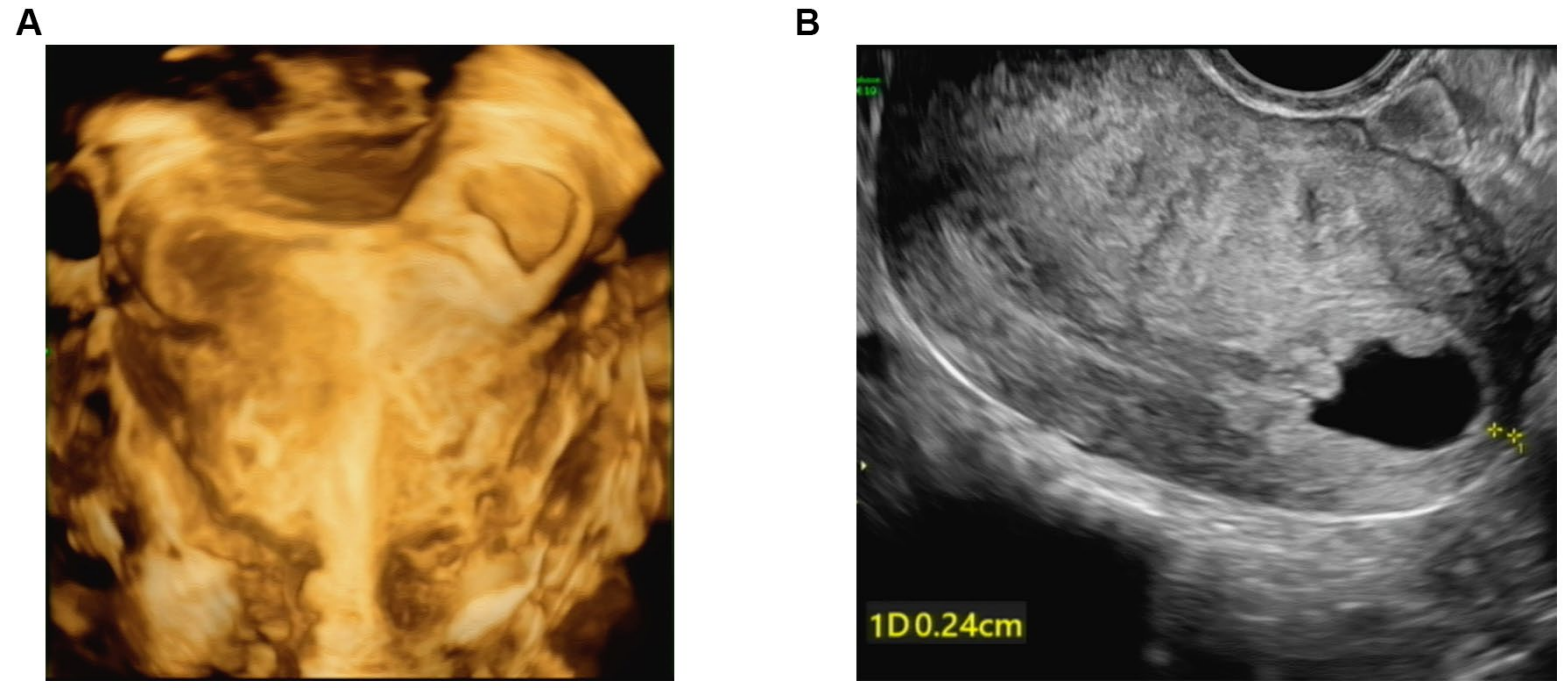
Ultrasonography images of angular pregnancy. (A) Three-dimensional ultrasonography image of an angular pregnancy with implantation medial to the uterotubal junction. (B) Two-dimensional ultrasonography image of an angular pregnancy with a myometrial thickness of 0.24 cm.
